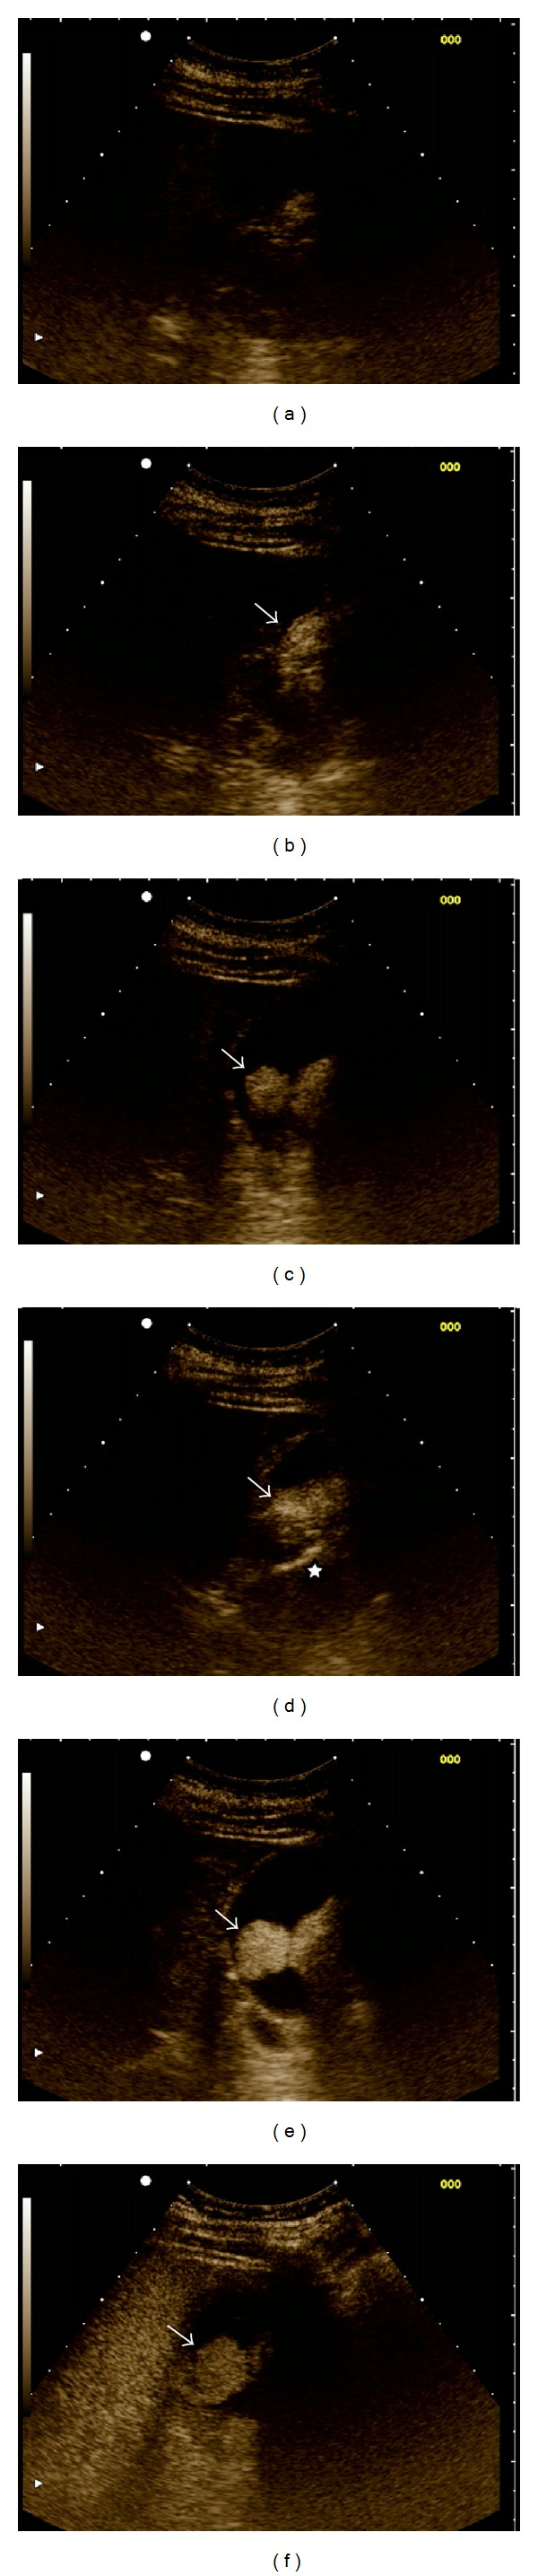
Contrast enhanced ultrasound (CEUS) of the gallbladder at various timepoints of contrast injection: (a) 0 sec (baseline); (b) 15 sec; (c) 23 sec; (d) 26 sec; (e) 35 sec; (f) 120 sec. The ultrasound monitor was set to sepia color mode for better contrast visualization. The lobulated gallbladder lesion shows intense and prolonged contrast enhancement (arrow). A feeding arterial vessel is shown in (d) (asterisk). See Supplementary Video Sequence.
